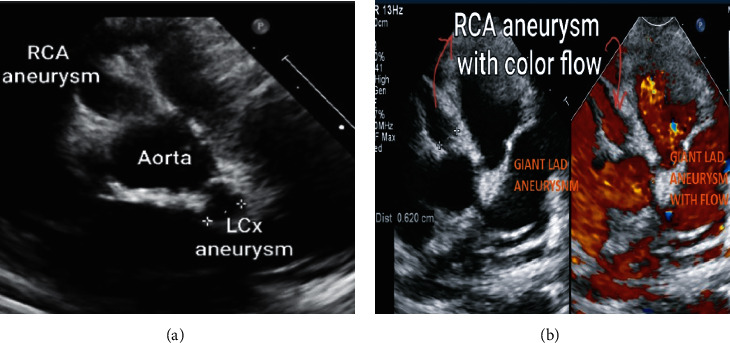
(a) Echocardiography still image showing left and right coronary aneurysms. (b) Echocardiography with color flow still image showing left and right coronary aneurysms.
